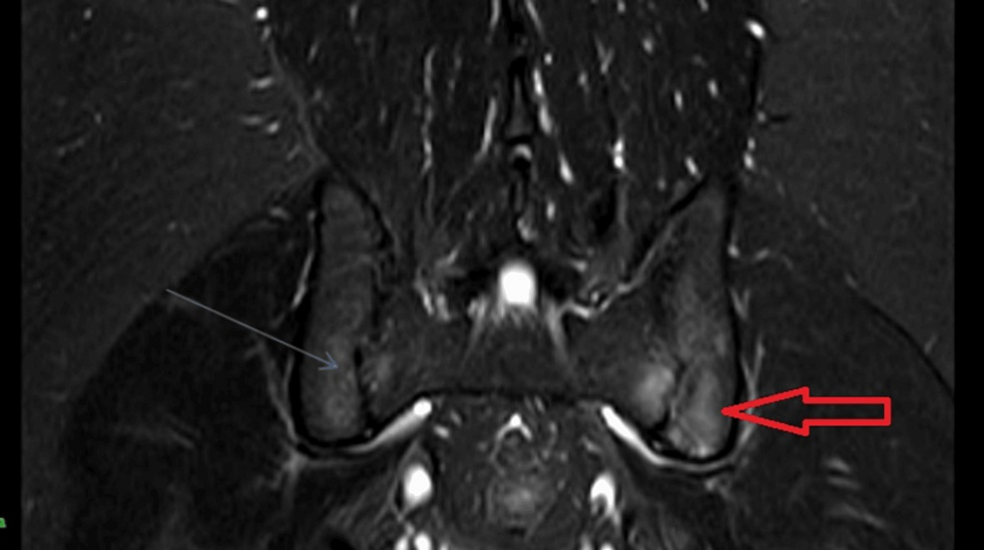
MRI (STIR image) of the pelvis showed bilateral sacroiliitis (left > right). STIR: short tau inversion recovery.
